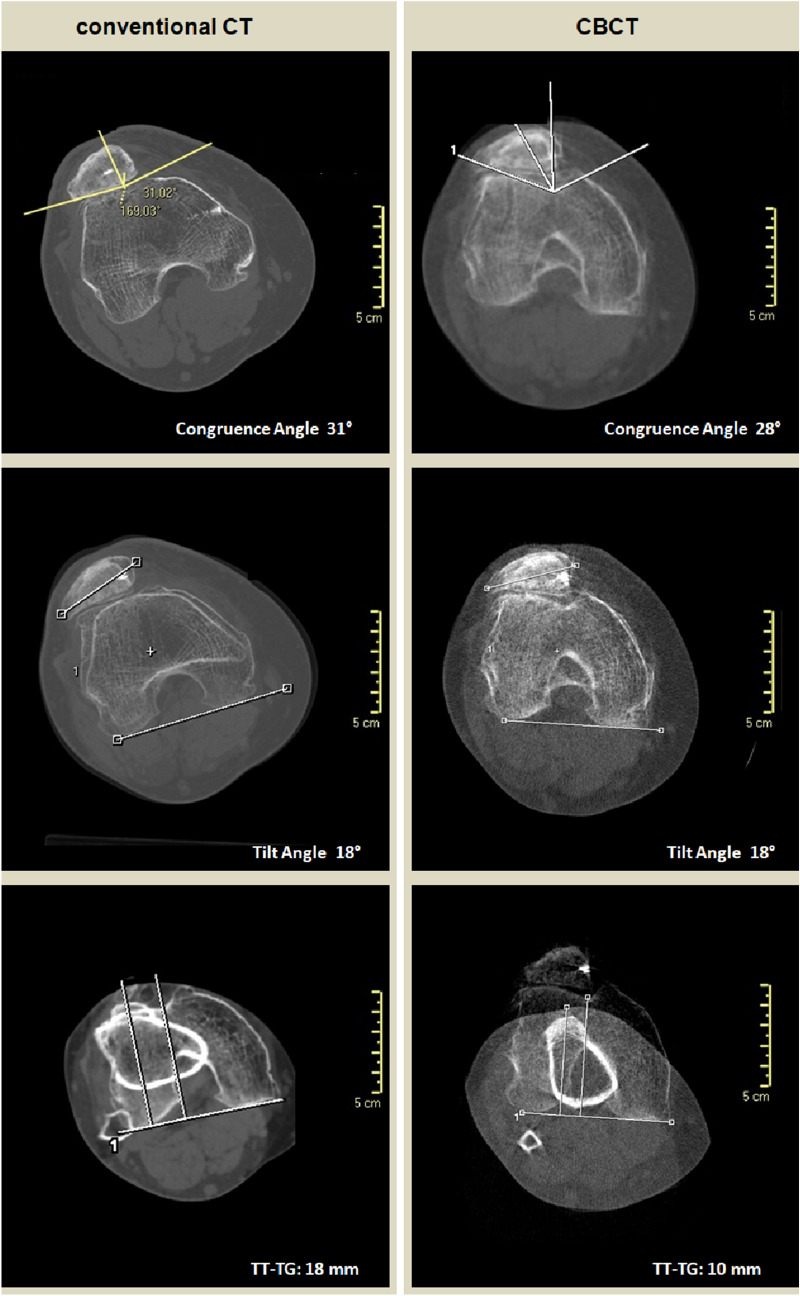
Measurements of patellofemoral stability and alignment on conventional computer tomography (CT) images and weight-bearing cone-beam CT (CBCT) performed on a single patient
